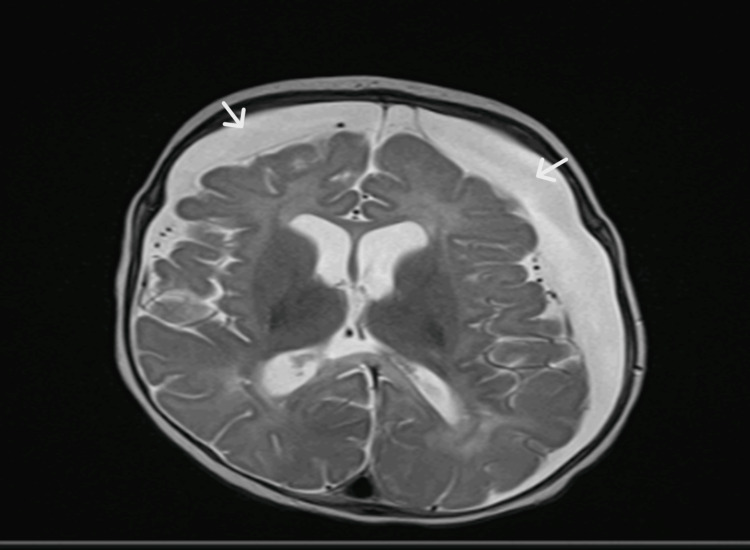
First brain MRI showing the bilateral subdural collections (white arrows) that caused a midline shift
